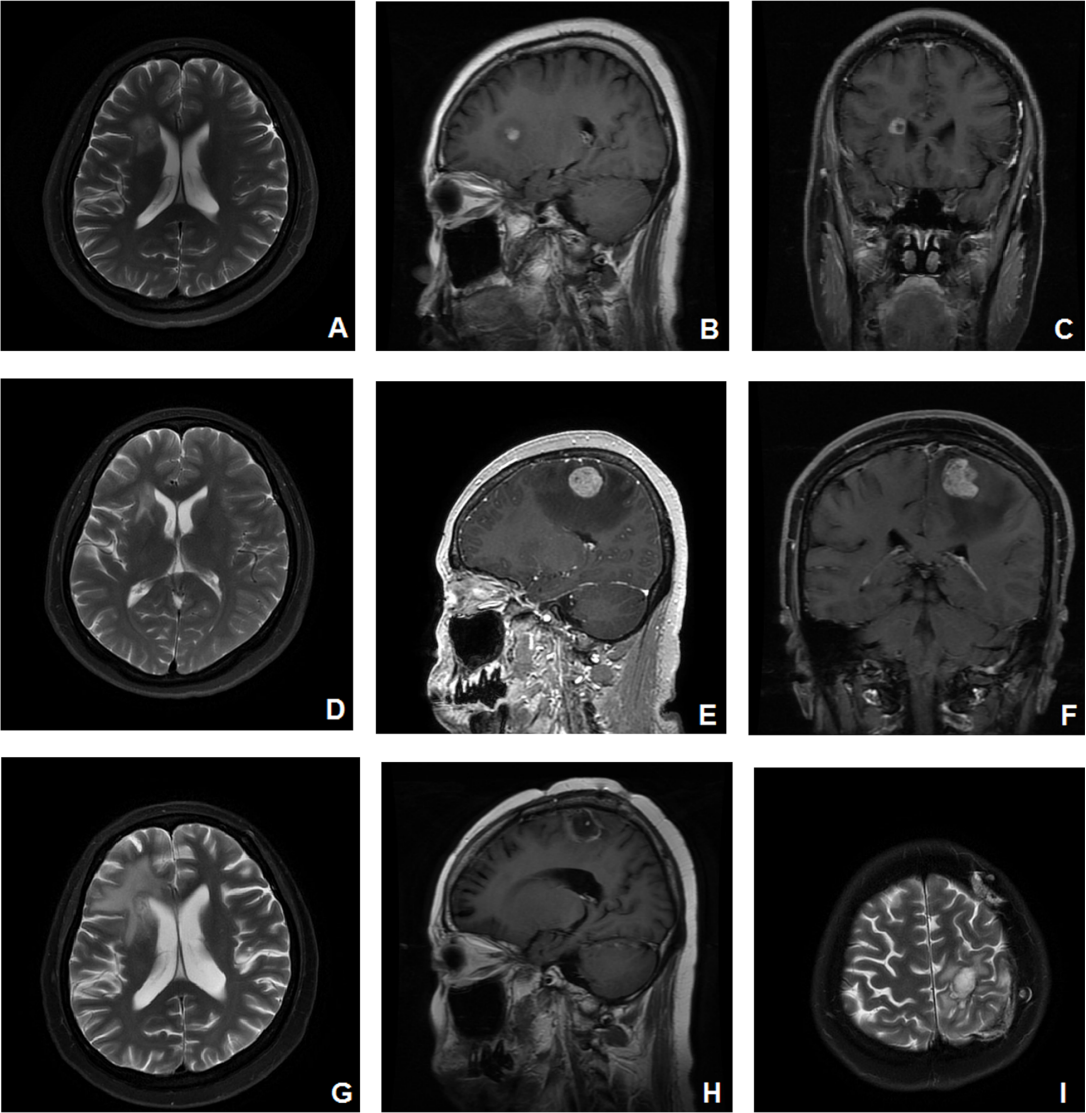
A preoperative MRI scan of the brain on November 18, 2016, showed a solitary mass in the right frontal lobe (a–c). One year later on December 25, 2017, a preoperative MRI scan of the brain showed multiple lesions in the bilateral frontal-parietal lobe (d–f). Three months after the operation, an MRI scan of the brain performed on May 3, 2018, showed postoperative changes in the bilateral frontal-parietal lobe, with gross total resection of the lesions (g–i). The MRI sequences consisted of the following: T2WI for a, d, g, and i; DWI for b, c, e, and f; and T1WI for h
